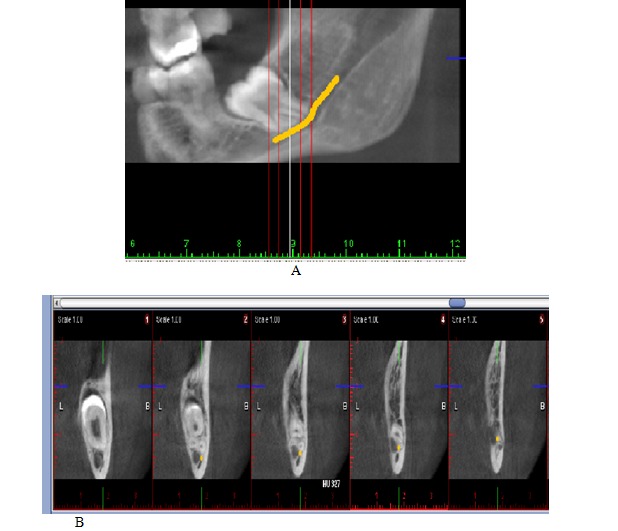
CBCT of left third molar: (A) 2D image; (B) multiplanar image.
